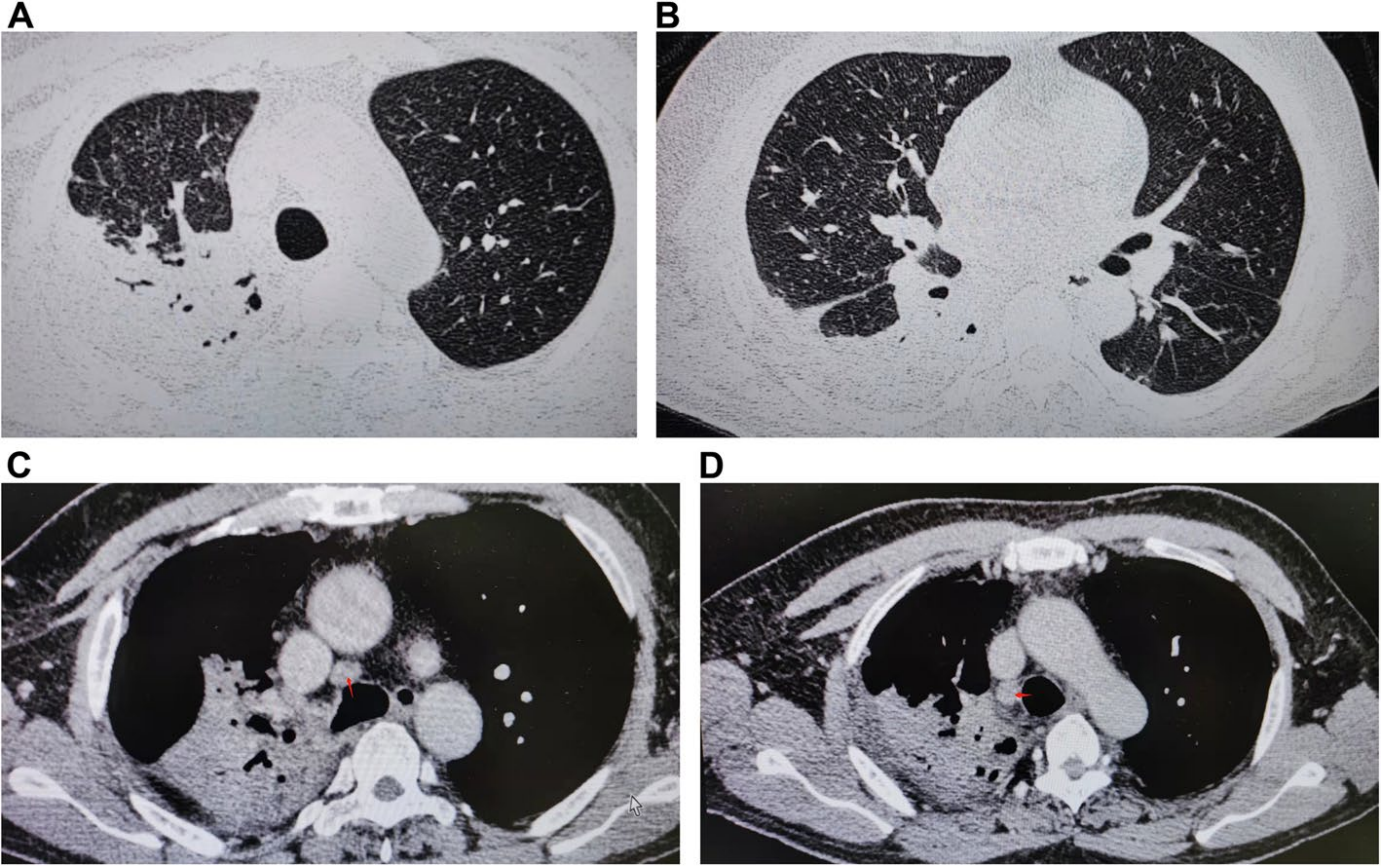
A–D Chest CT image showing patchy infiltration in the right upper lobe and lower lobe in the lung window, as well as lymph node enlargement in the mediastinal window (July 2016). CT, computed tomography
